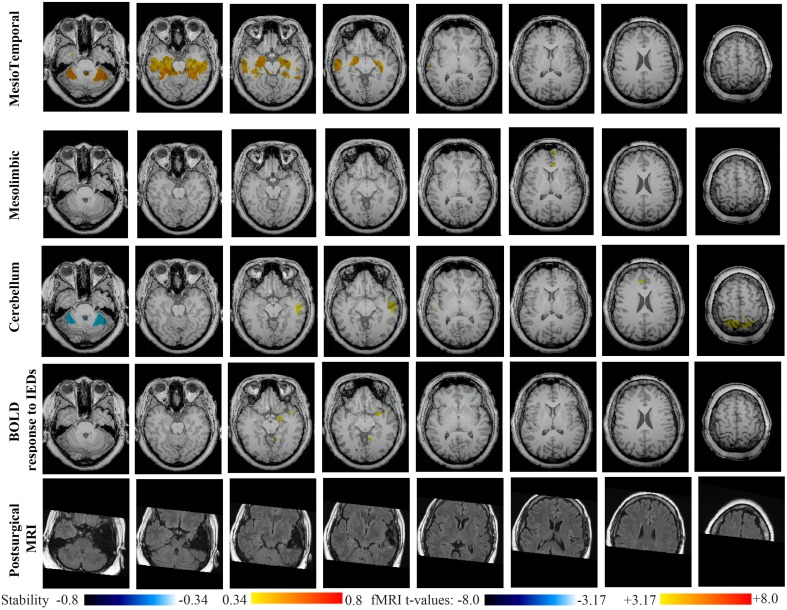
Evaluation of DANI on Patient 4 with right MTLE. Results are presenting most salient stability changes observed in *Cmap*_*n*_ followed by a *t*-map of the BOLD response to epileptic discharges and a postsurgical FLAIR MRI, all resampled in the native anatomical MRI space of the patient. Most salient stability changes observed for *Cmap*_*n*_ of Mesio-Temporal and Mesolimbic networks are presented. We also present *Cmap*_*n*_ of the Cerebellum network (max. stability increase of 0.48), since it was involved in significant interactions of modularity changes at *p* < 0.001. Same colormap conventions than in Figure 6.
